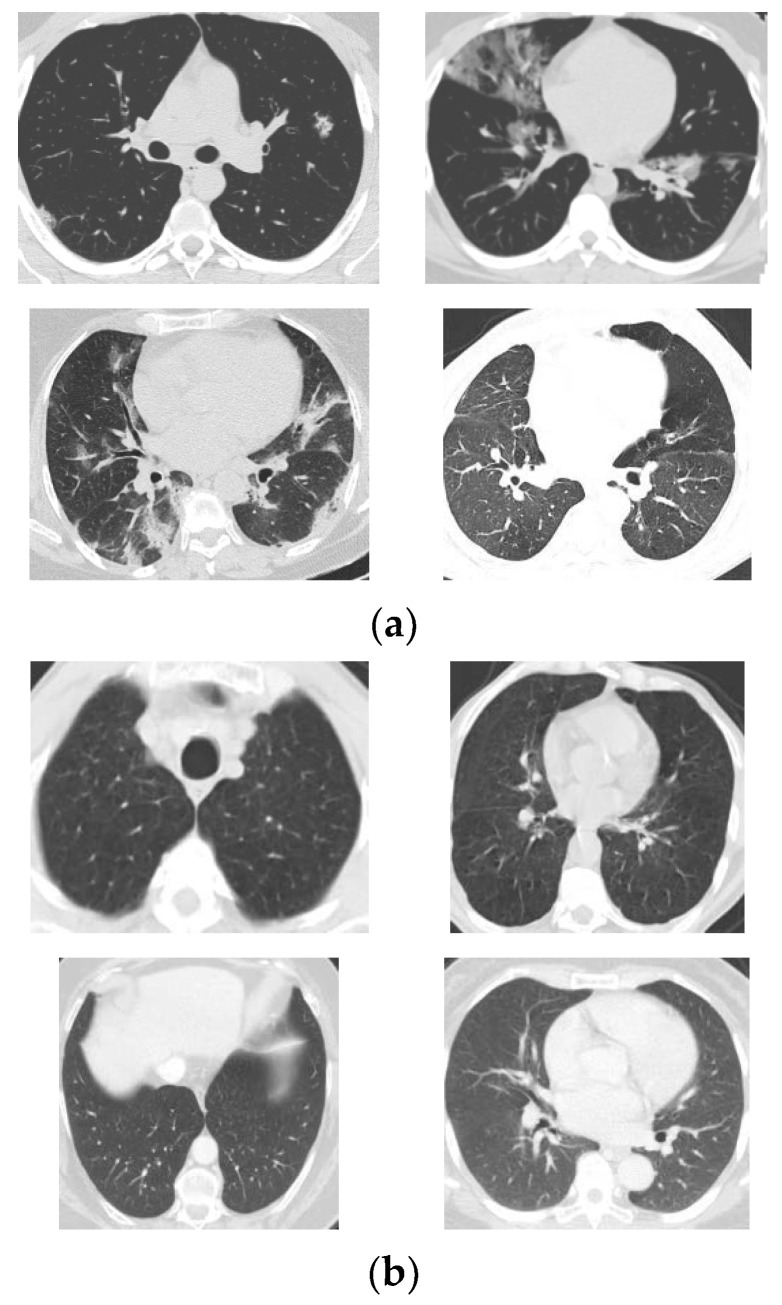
Random CT scans in the SARS-CoV-2 dataset ((a) COVID-19 samples, (b) Non-COVID-19 samples).
